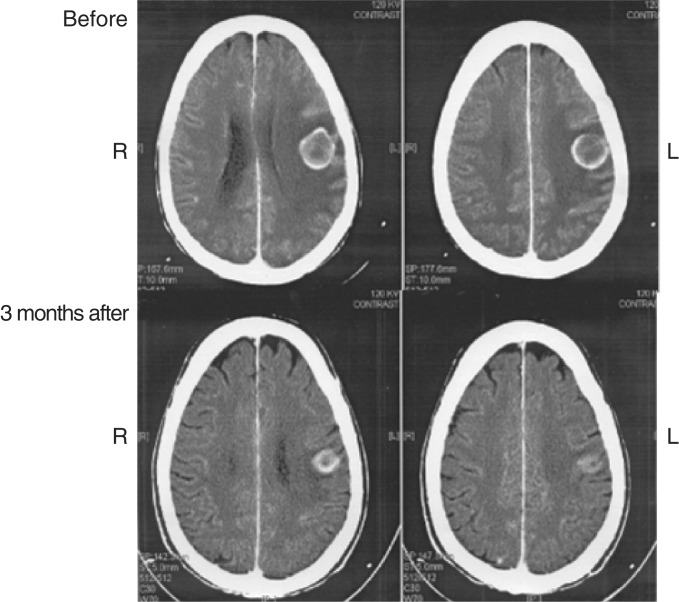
Computed tomography scan before and after treatment with gefitinib. Reproduced with permission from: Cappuzzo F, Calandri C, Bartolini S, Crino L (2003b).
